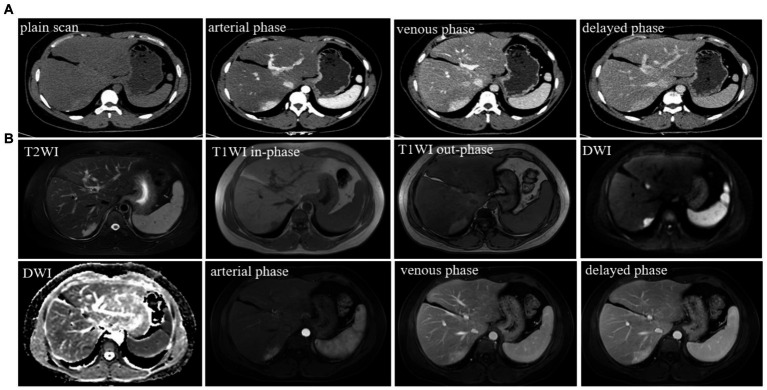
(A) Typical level and imaging findings of abdominal CT images for the recurrent lesion in the S7 segment of the liver; (B) abdominal MR typical images for the recurrent lesion in the S7 and S8 segment of the liver.
